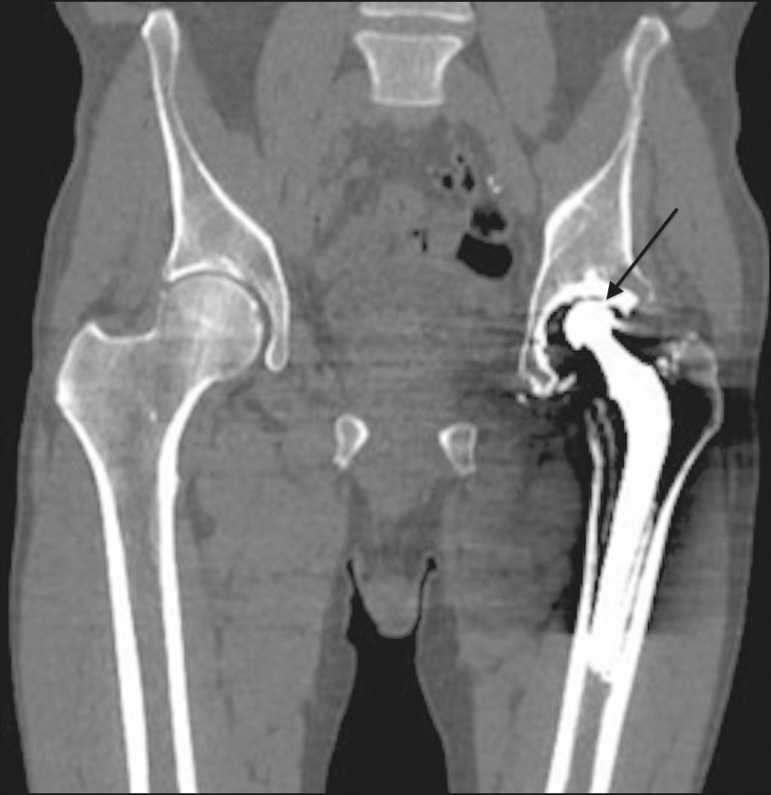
Coronal computed tomography scan showing a total left hip prosthesis, with asymmetry of the upper joint space, suggestive of wear of the polyethylene lining (arrow).
